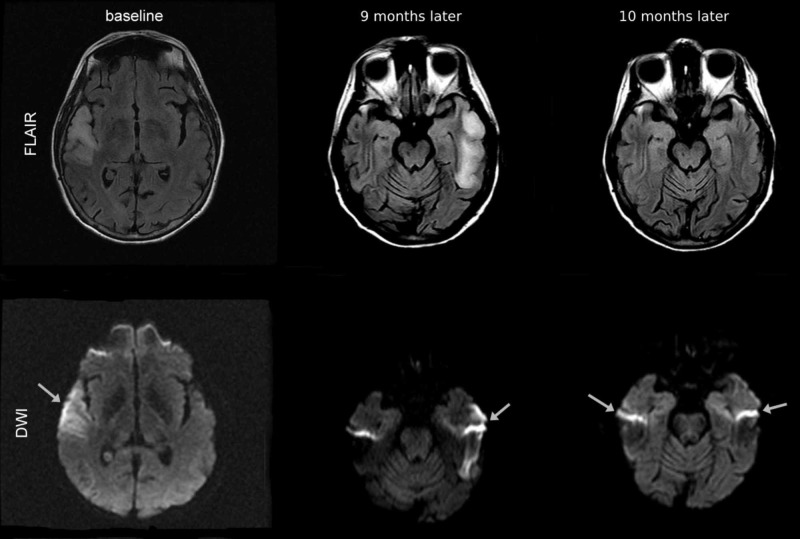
MRI FLAIR and DWI images at first presentation and until 10 months later are presented. FLAIR images demonstrate step-wise progressing FLAIR hyperintense edema not corresponding to a vascular territory. DWI delineates increased signal (T2 shine-through), as shown by the arrows. MRI, magnetic resonance imaging; FLAIR, fluid-attenuated inversion recovery; DWI, diffusion-weighted imaging
